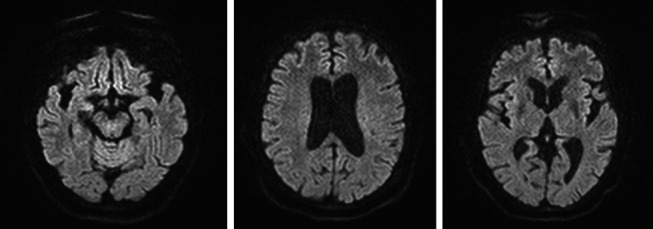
DWI sequence with various slices shows no diffusion restriction.
